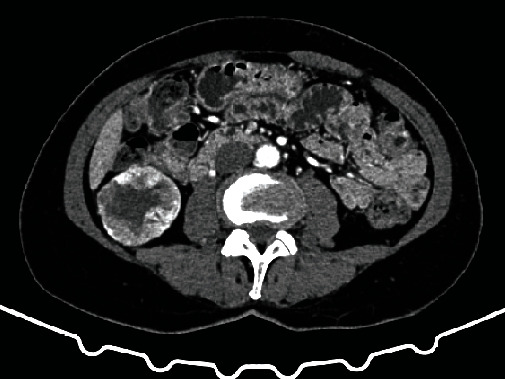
Computed tomography showed a solid mass measuring 40 × 36 × 30 mm in the right kidney.
